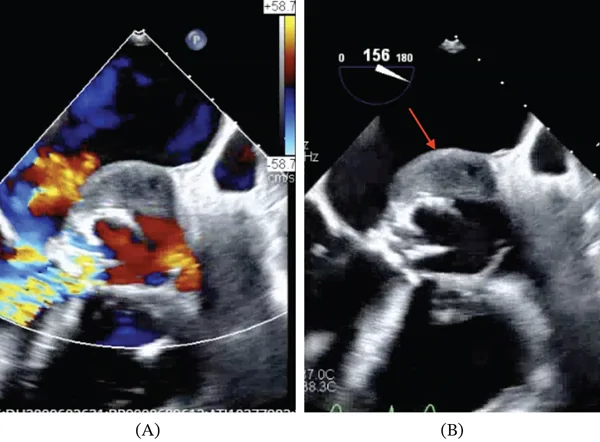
(A) Color Doppler transesophageal echocardiography demonstrating a large eccentric paravalvular regurgitant jet originating adjacent to the bioprosthetic valve annulus, consistent with prosthetic valve dehiscence and periannular destruction. (B) Transesophageal echocardiography demonstrating distortion of the bioprosthetic valve architecture with annular involvement, concerning for periannular abscess and prosthetic instability.
